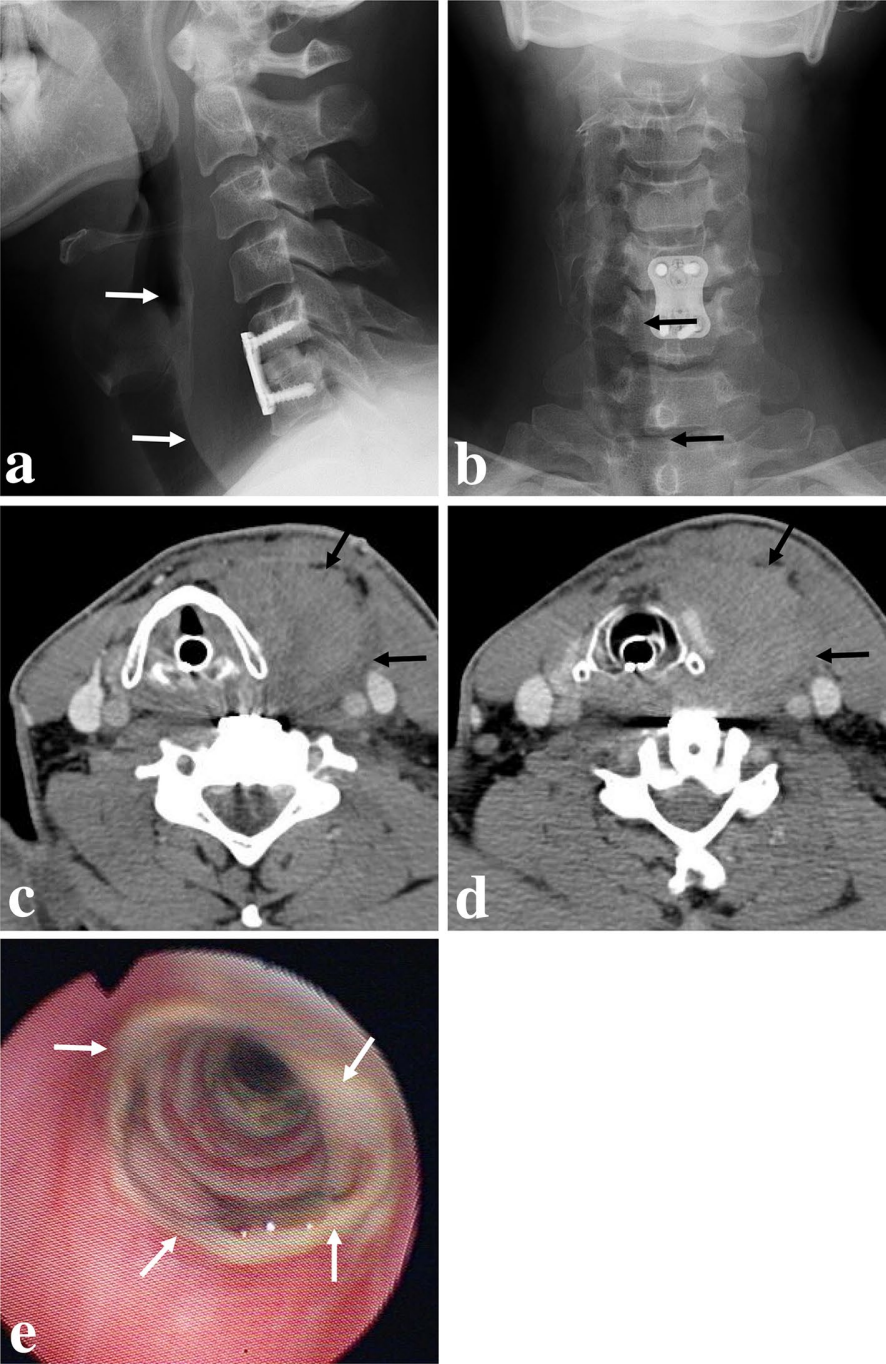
Seven days after surgery, follow-up lateral (a) and anteroposterior (b) radiographs showed increased anterior soft tissue swelling (white arrows) and tracheal deviation to right side (dark arrows). Neck-enhanced computed tomography scans (c and d) showing anterior and right lateral displacement of the airway including the trachea by a large retropharyngeal hematoma (dark arrows). Laryngoscopy (e) showed tracheal granulation tissues (white arrows)
